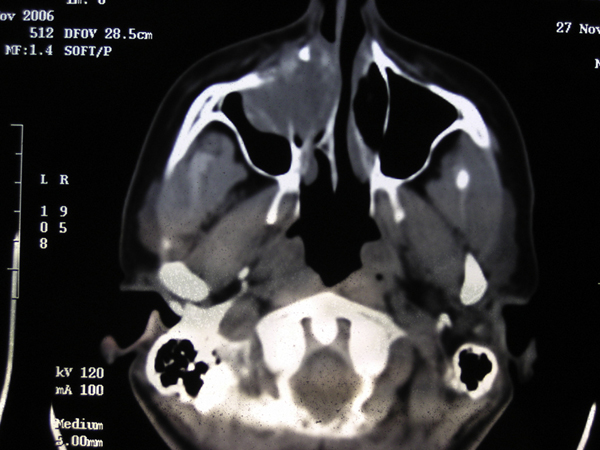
Axial computed tomography of the paranasal sinuses showing a round osteolytic lesion of the right maxillary sinus.
